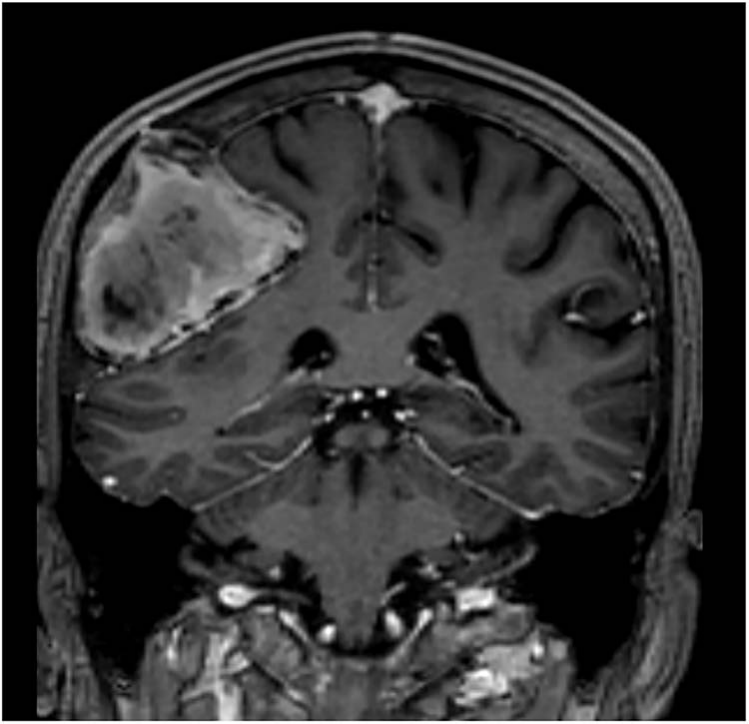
T1-weighted contrast-enhanced coronal MRI scan showing the lesion with intralesional haemorrhagic products. There is no evidence of dural invasion.
